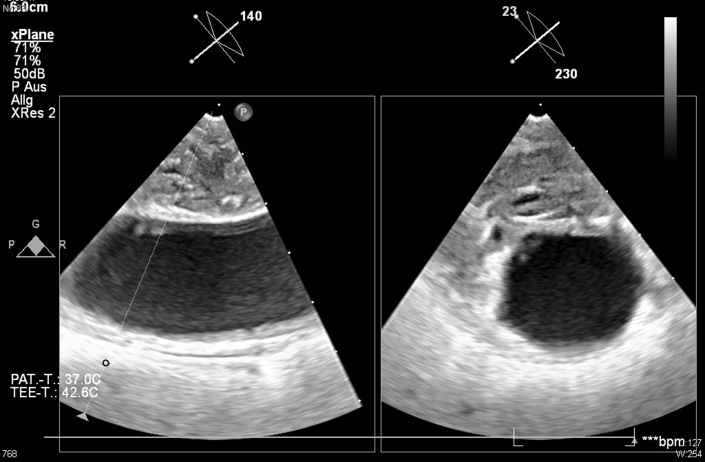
Transesophageal Echocardiography After Mass Removal
